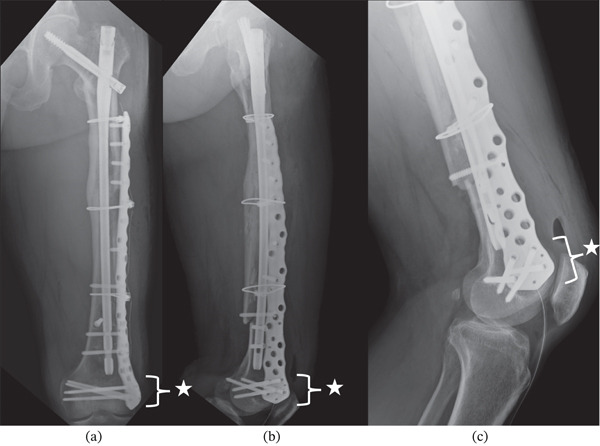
Plain radiographs taken after the patient′s second surgery. (a, b) Anteroposterior and lateral views, showing maintained femoral alignment from the first surgery, with additional plate fixation. (c) Multiple screws were fixed to the distal femur, providing sufficient stability to the femoral condylar fragment. Stars indicate additional stabilizing screws from the second‐stage periprosthetic plate.
